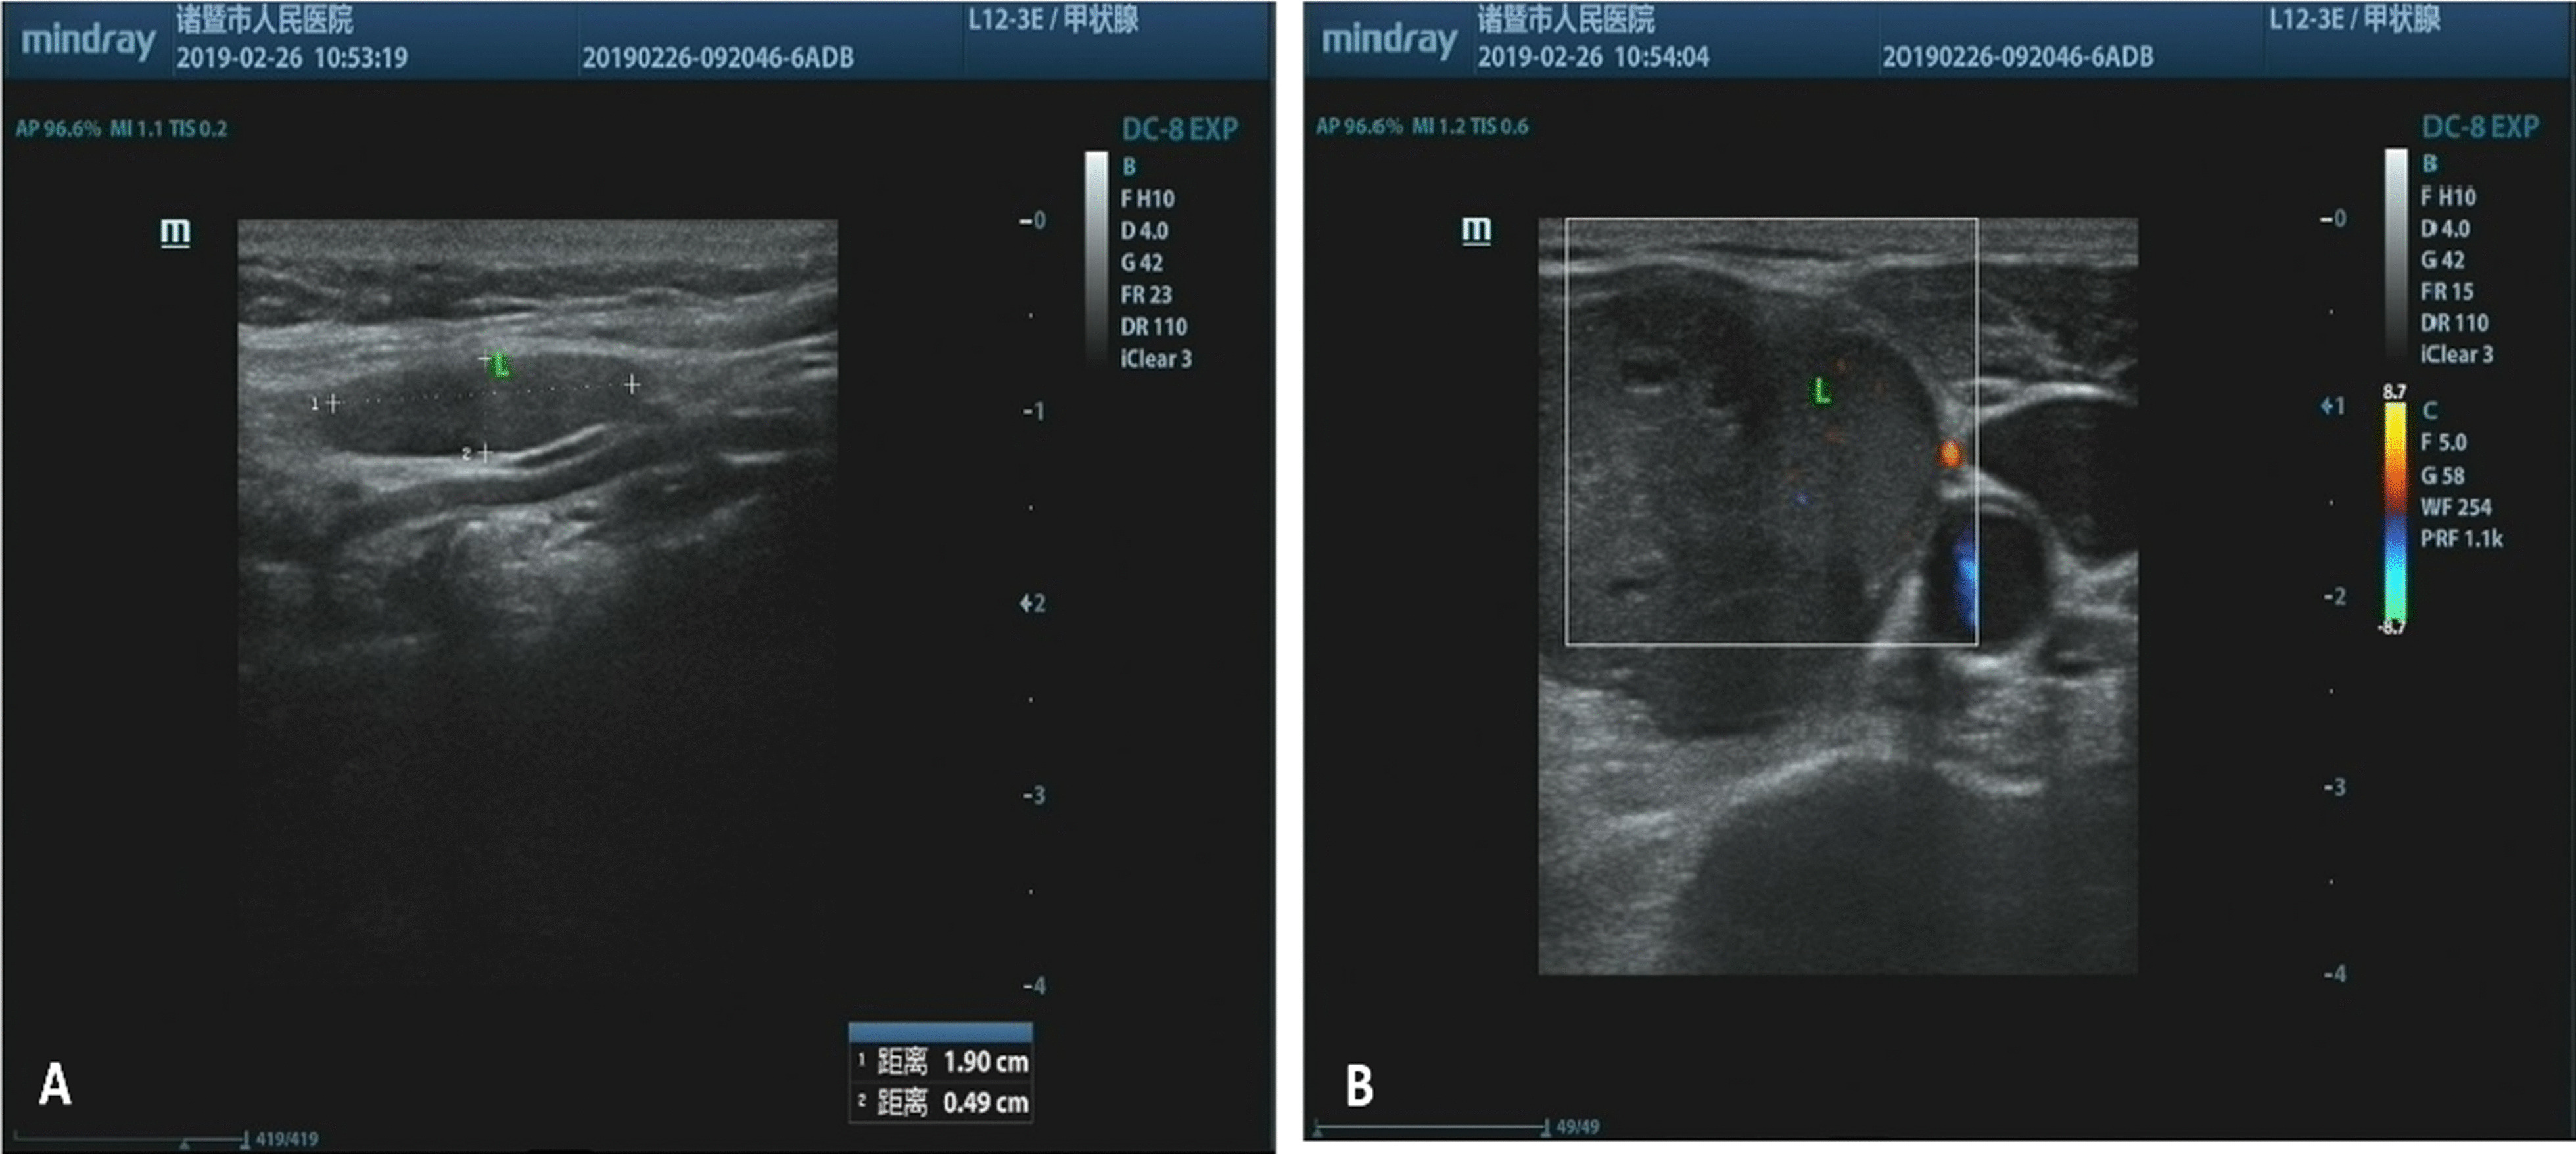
Neck ultrasonography showing an elliptical tumor with soft tissue density and ill-defined boundary adhered to the left lobe of the thyroid (A and B)
